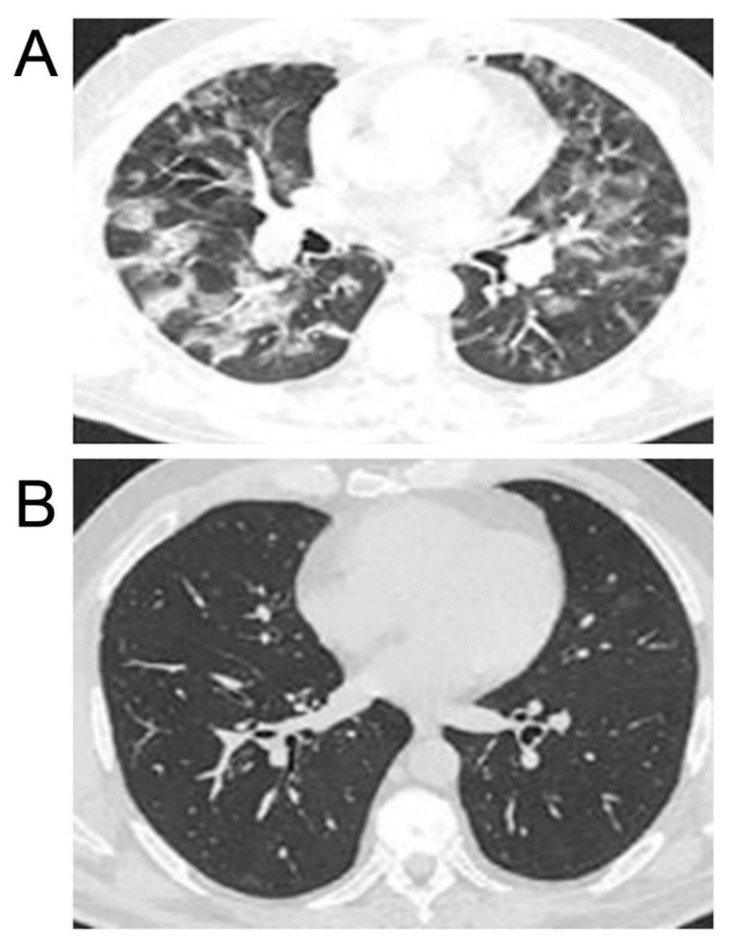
The results of computer tomography (CT) of the lungs of COVID-19 patients. (A) Severe case. 80% lung injury before STS treatment. (B) 5% lung injury after 2.5 months of application of hot helium–oxygen mixtures and inhalation of STS.
